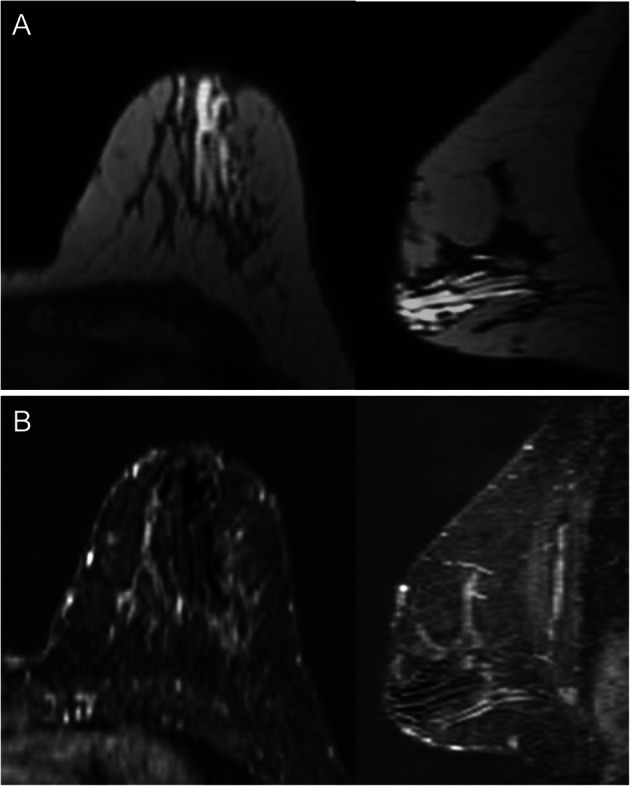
Ectasia and MRI. Screening MRI in an asymptomatic high-risk 38-year woman 6 months after lactation. a Unenhanced axial and sagittal T1-weighted images. b Contrast-enhanced axial and sagittal T1-weighted MRI (subtracted image obtained 120 s after contrast injection). Note the tubular structures in the retroareolar region of the left breast with a segmental distribution; proteinaceous material causes increased signal intensity on T1-weighted sequences, but not intraductal enhancement
